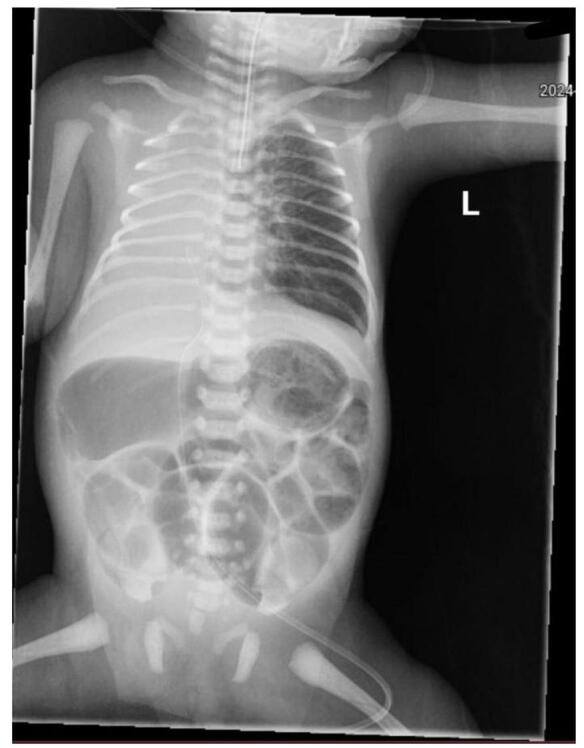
Anteroposterior (AP) plain abdominal radiograph demonstrating a disproportionately dilated loop of colon proximal to the site of atresia, with a notable transition zone and air-fluid levels, consistent with mechanical obstruction. The distal colon appears collapsed, and there is no evidence of pneumoperitoneum or abnormal calcifications.
